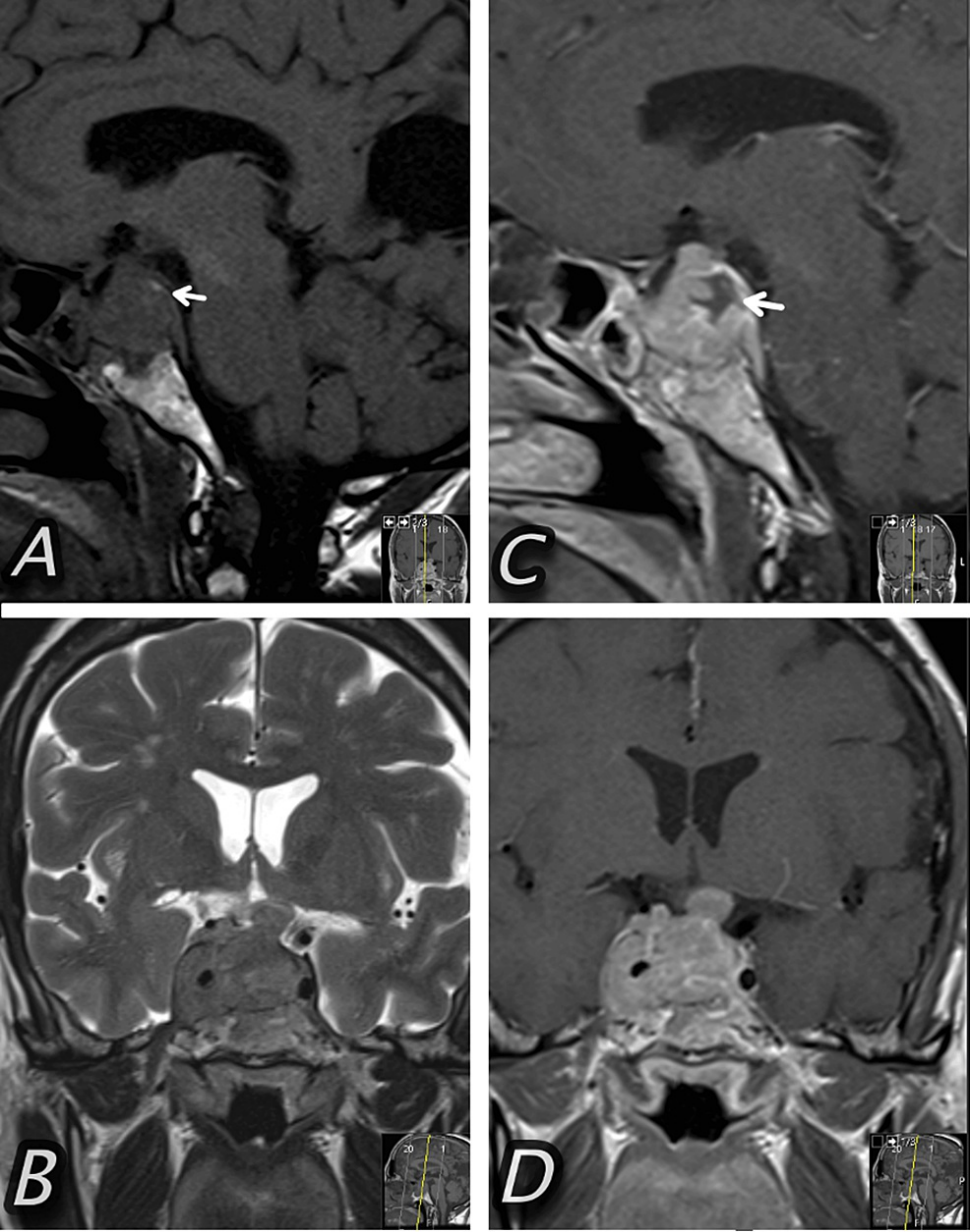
Brain MRI. (A) Sagittal T1-weighted image (WI) showing the large sellar lesion and the induced bone remodeling of the sphenoid; a small region of a high-intensity signal within the mass (small arrow) is seen that could correspond to blood products. (B) Coronal T2 WI showing a lobulation in the cranial aspect of the lesion that compresses the optic chiasm (not present in the previous MRI); it also shows an invasion of the right cavernous sinus. (C and D) Contrast-enhanced T1 WI sagittal and coronal, respectively. The mass enhances after contrast and it is possible to define areas of necrotic/cystic degeneration (small arrow).
